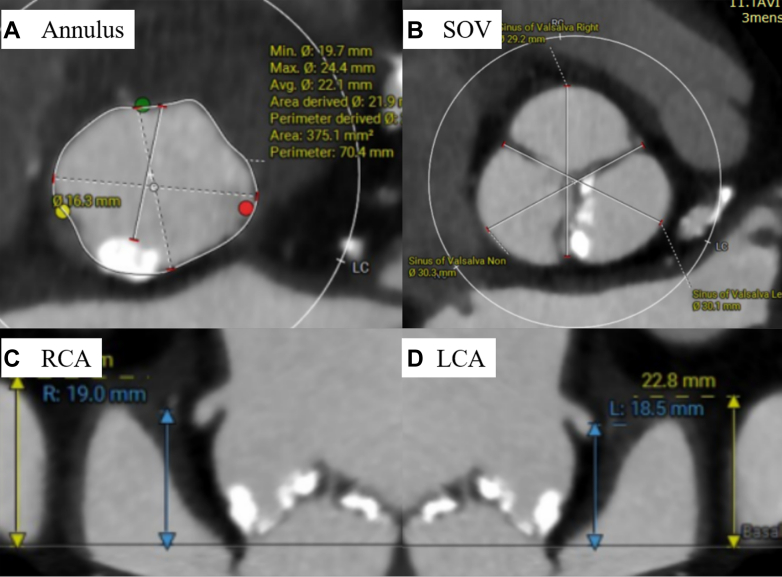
Preprocedural Computed Tomography Images of the Aortic Valve Complex Shown are images of the (A) annulus, (B) sinus of Valsalva (SOV), (C) right coronary artery (RCA), and (D) left coronary artery (LCA).
